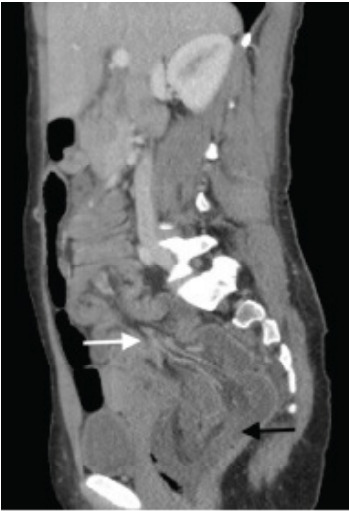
Sagittal computed tomography view showing edematous sigmoid colon (black arrow) containing omentum (white arrow).
